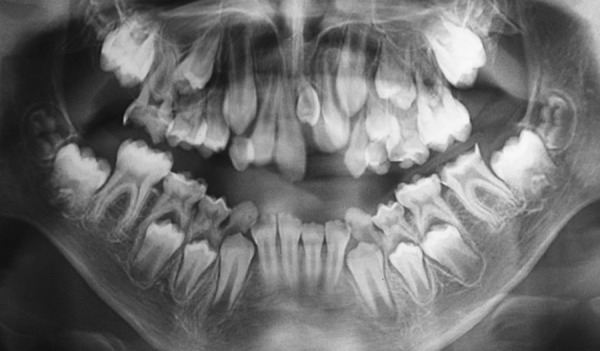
Panoramic radiograph attesting the presence of the supernumerary tooth in the anterior region of maxilla
